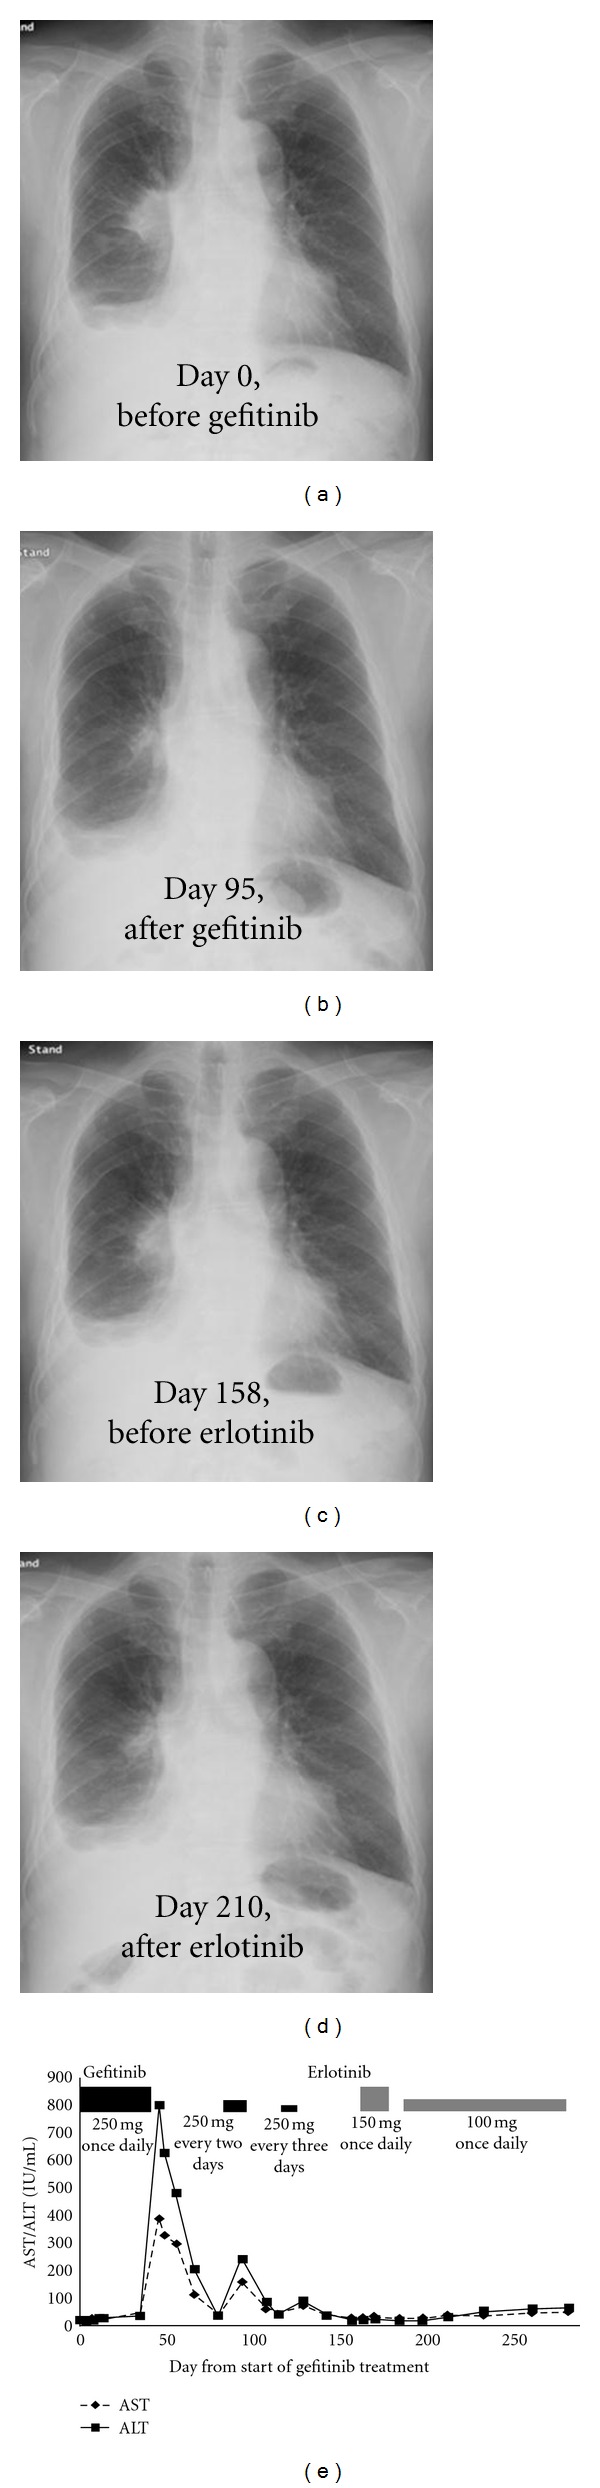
The course of chest X-ray images and transaminase levels during gefitinib and erlotinib treatments.
